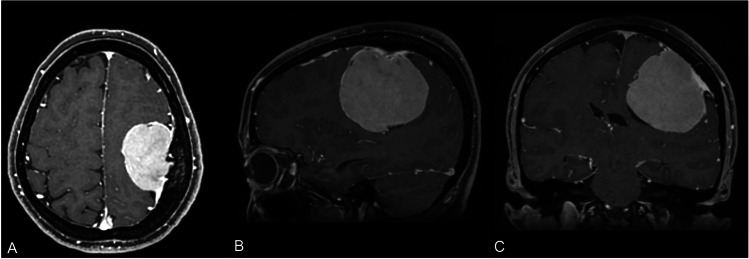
Contrast-enhanced brain MRI, axial (A), sagittal (B) and coronal (C) TI-weighted sequences: large (6 cm) left rolandic meningioma with radiologic findings of benign (WHO grade I) histotype
